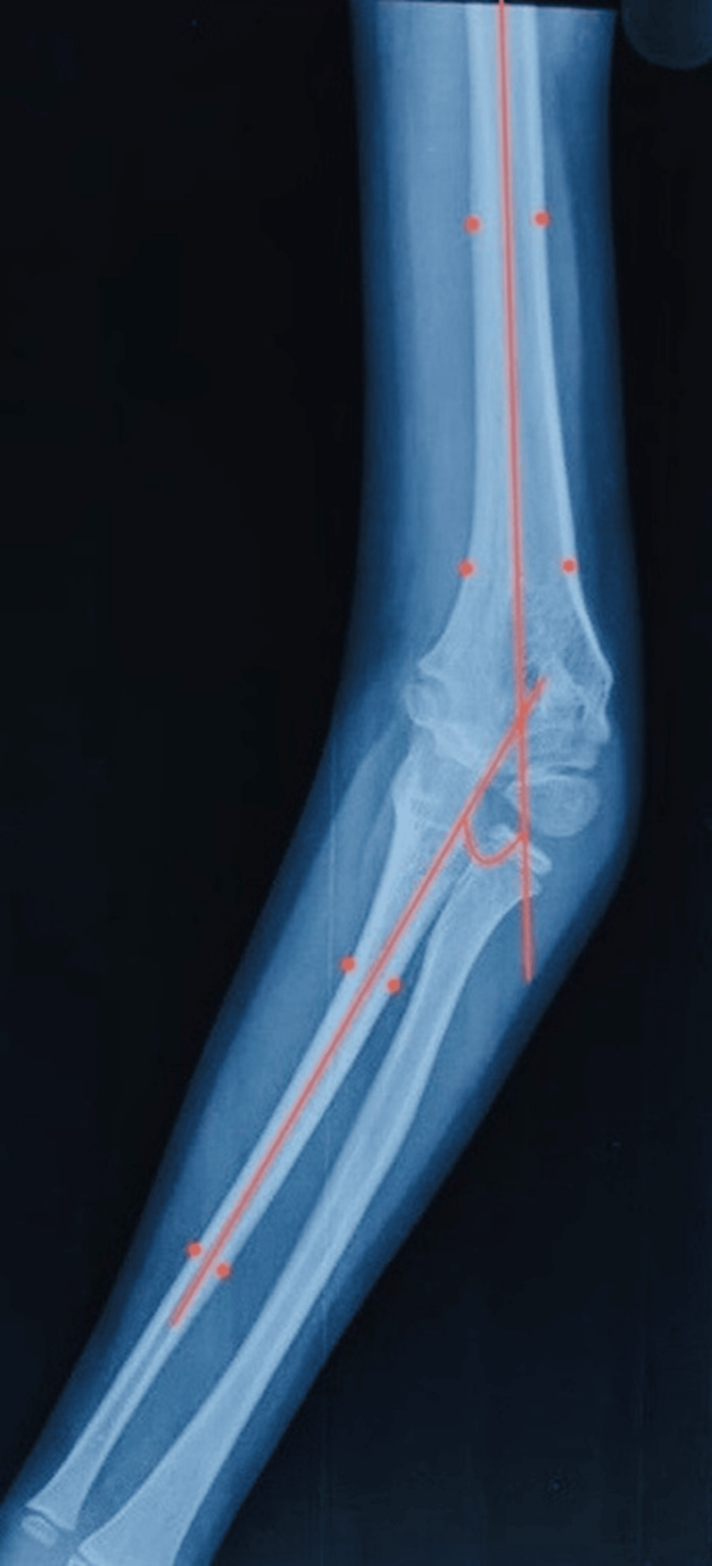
Radiograph showing HUA calculated as the angle between the mid-humeral and mid-ulnar axis HUA: Humero-ulnar angle
